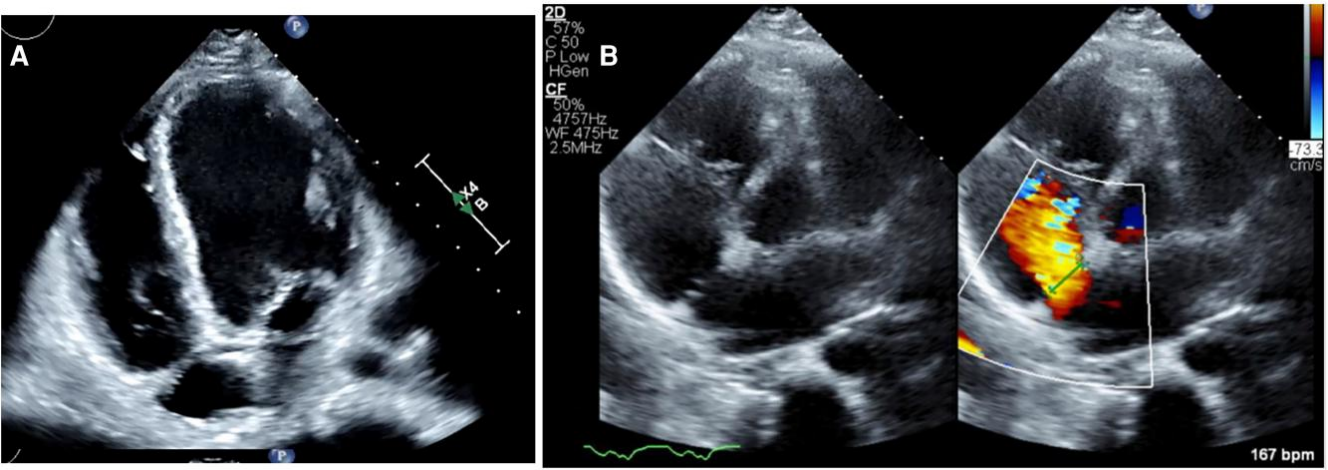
Transthoracic echocardiogram prior to commencing veno-arterial extracorporeal membrane oxygenation demonstrating (*A*) dilated left ventricle with thinned septum and apex on apical four-chamber view and (*B*) secundum atrial septal defect with left-to-right shunting with colour Doppler on parasternal short-axis view.
